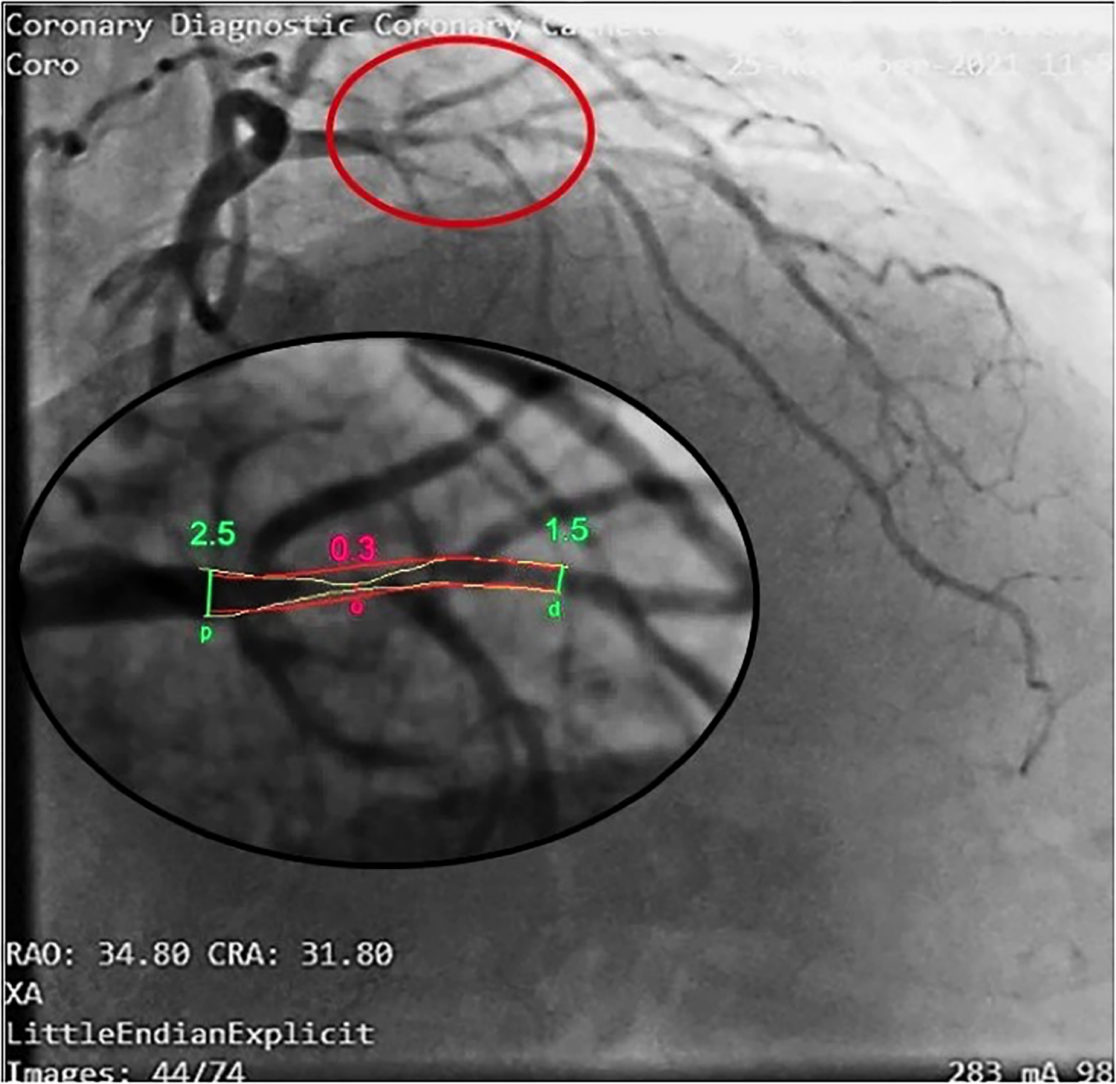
Coronary angiography in the same patient as in Figures 5, 6. In this right oblique view, the site of the stenosis (encircled in red) has been magnified (at the bottom) to measure the minimal and maximal diameters (QCA). Coronary angiography confirms a very tight stenosi almost occlusive of the proximal LAD (QCA = 98% area stenosis) confirming the E-Doppler TTE evaluation in terms of stenosis detection, location, and severity assessment. QCA, quantitative coronary angiography. LAD, left anterior descending coronary artery.
